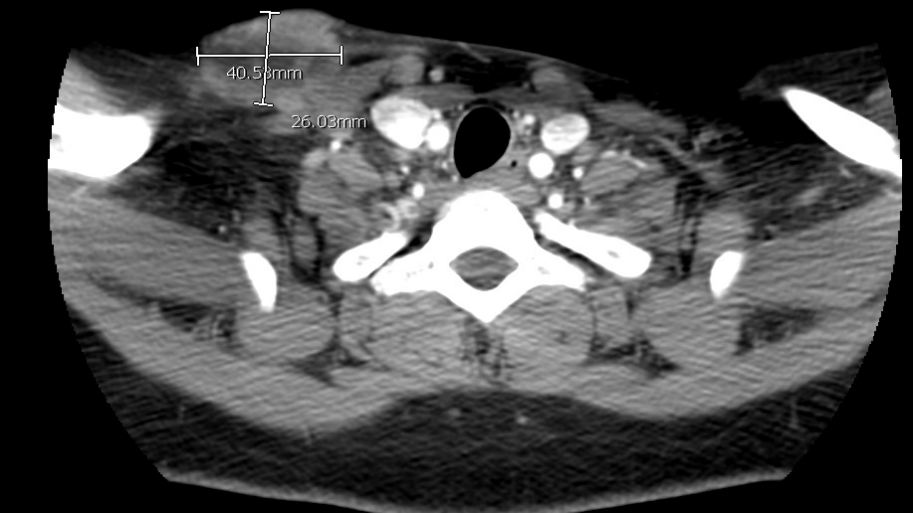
Computed tomography scan of the neck with supraclavicular mass
